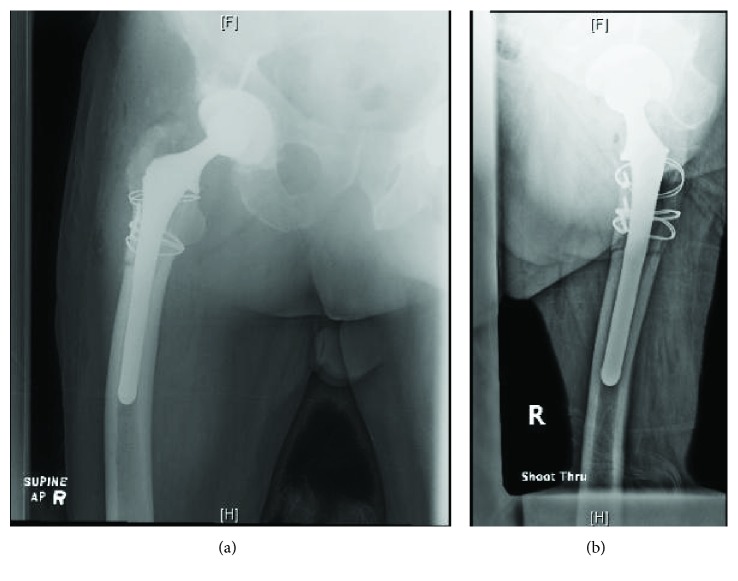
(a) Anterior-posterior radiograph demonstrating stable fixation after revision surgery. (b) Lateral radiograph demonstrating the same postrevision fixation.
